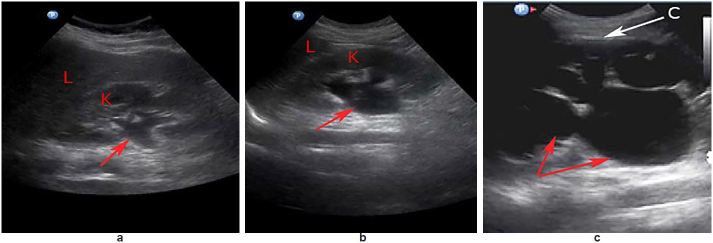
Hydronephrosis visualized as an anechoic black area on ultrasound. Figure 1a: mild hydronephrosis (red arrow) showing dilation of the proximal renal pelvis of the kidney (K), liver (L); Figure 1b: moderate hydronephrosis (red arrow) showing dilation of the renal pelvis and calyces of the kidney (K), liver (L); Figure 1c: severe hydronephrosis showing large dilation of the renal pelvis and calyces (red arrows) extending outward and resulting in a thinning of the renal cortex (C).
